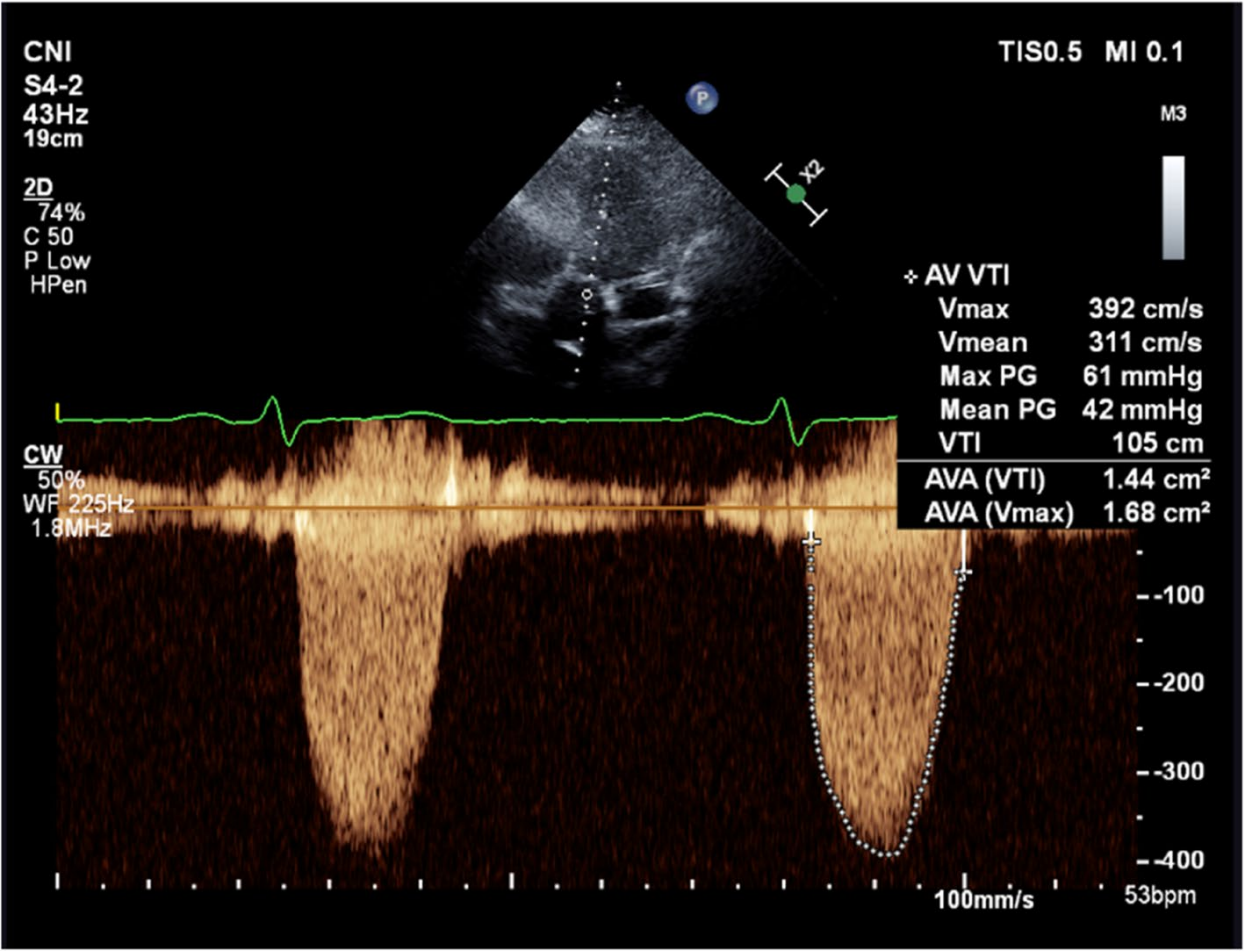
Transthoracic echocardiography prior to clinical presentation. Elevated aortic valve hemodynamic on transthoracic echocardiography 2.5 years following surgery
